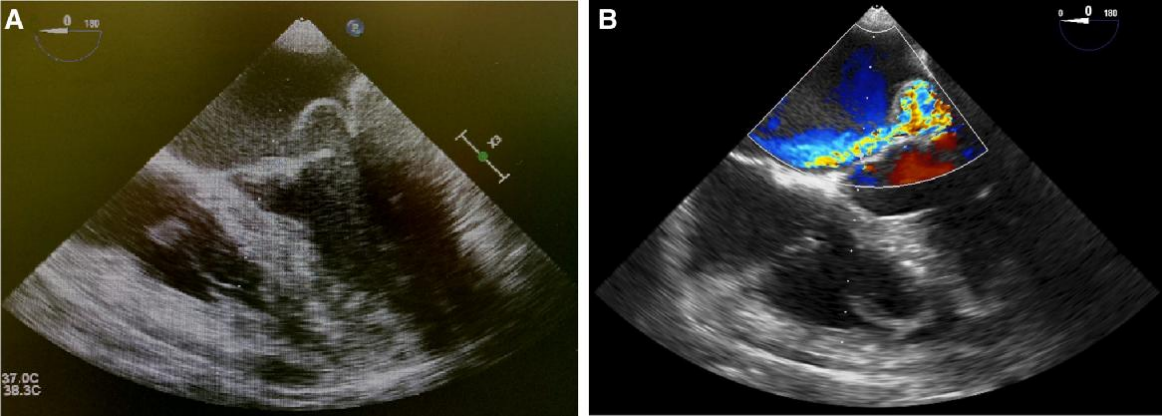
(*A* and *B*) Peri-operative transoesophageal echocardiography mid-oesophageal four-chamber (ME 4C) view showing severe eccentric mitral regurgitation with prolapsed P2 scallop causing Coanda effect.
